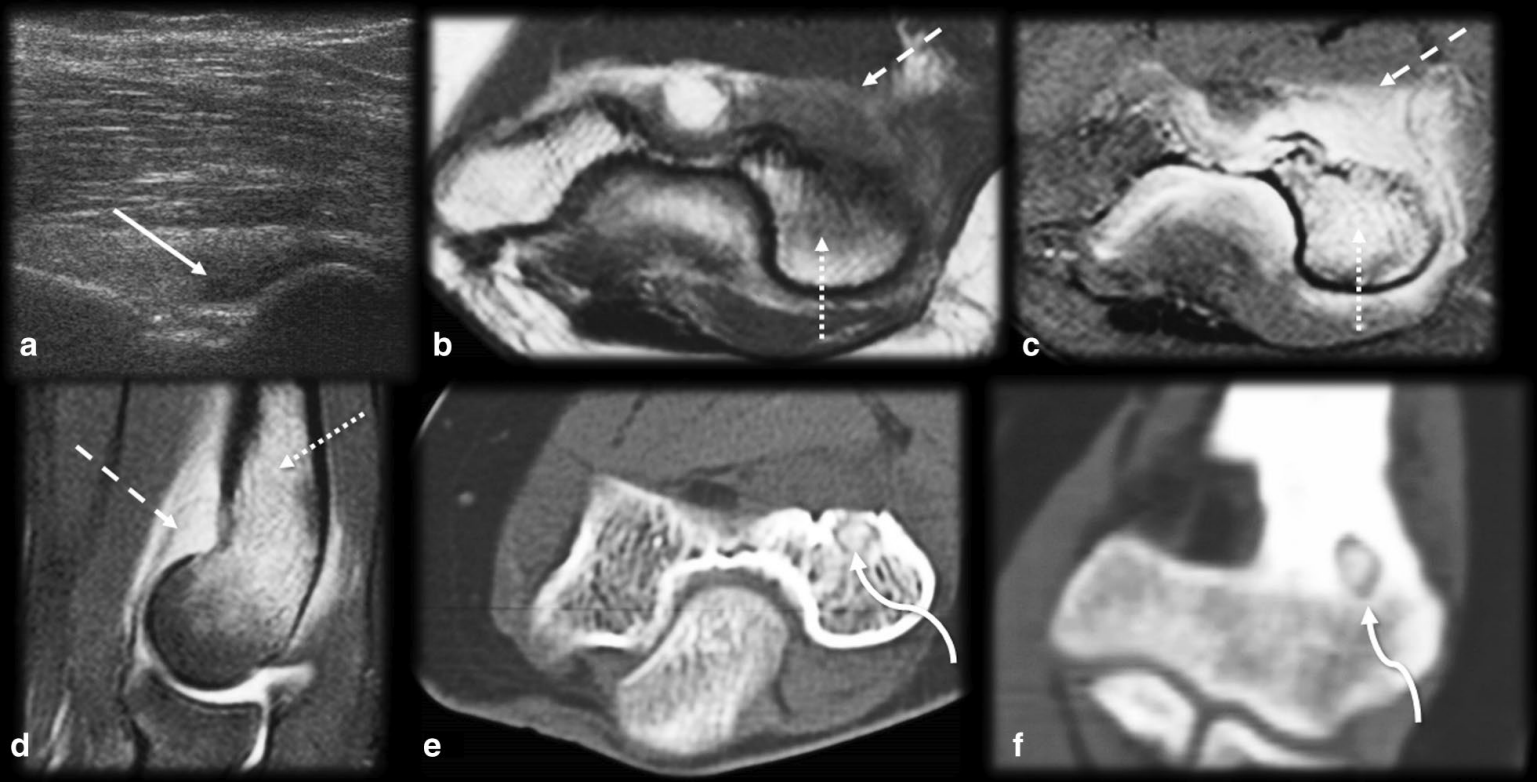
Osteoid osteoma mimicking synovitis on the elbow. Female, 20 years old, with elbow pain and edema for 4 months. Ultrasound in anterior sagittal view (a) at the coronoid fossa level shows joint effusion (arrow). MRI T1 (b) and T2FS (c, d) show bone marrow edema (dotted arrows) and synovitis (dashed arrows). Further investigation with a CT scan (e, f) revealed a mineralized nidus (curved arrow) in the cancellous bone of the medial humeral condyle
